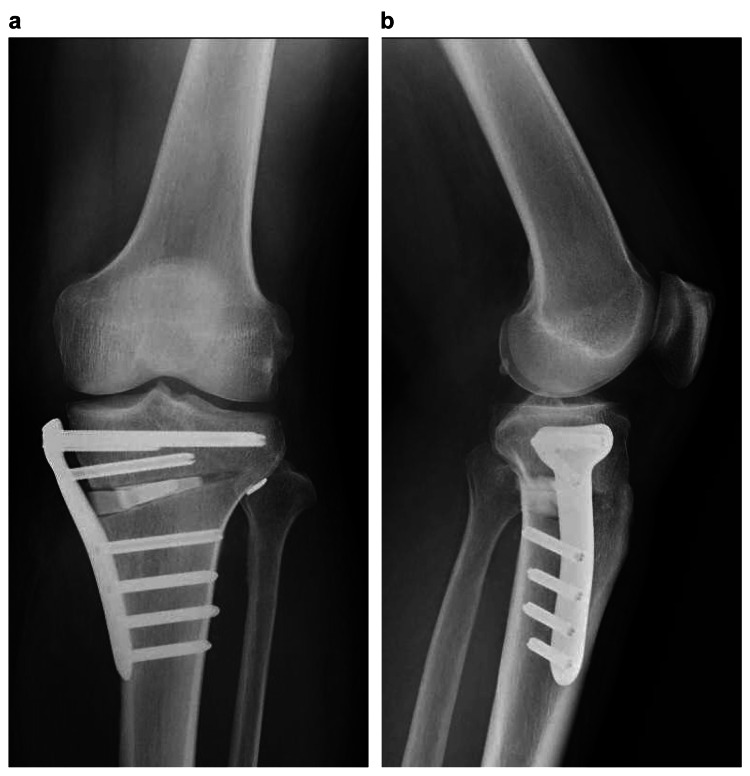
Postoperative plain radiography (a) frontal view; (b) lateral view
